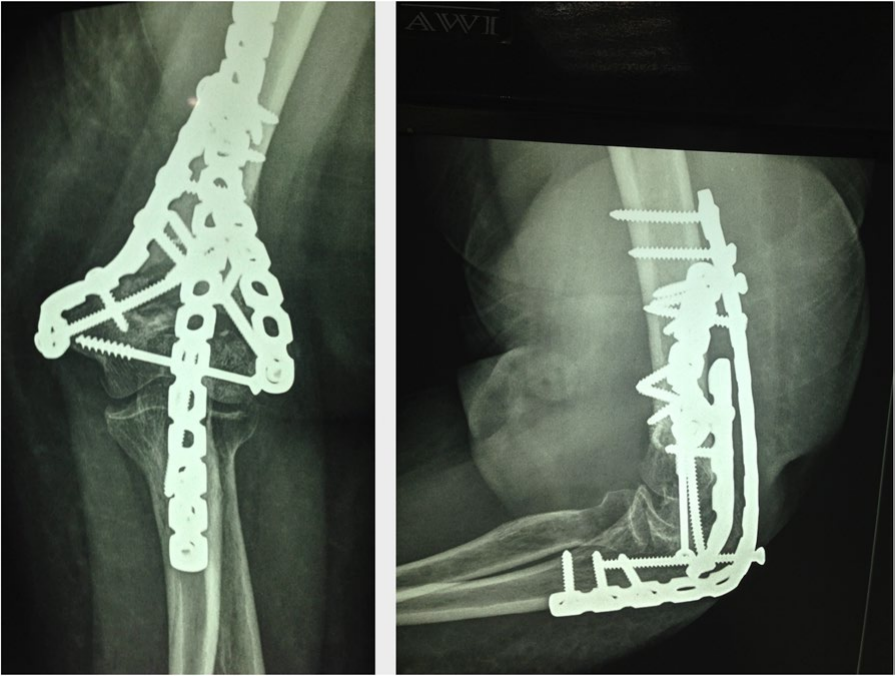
Postoperative x-rays of the forementioned patient (case #9) fixed with double columns plates and augmented with a temporary spanning elbow plate
